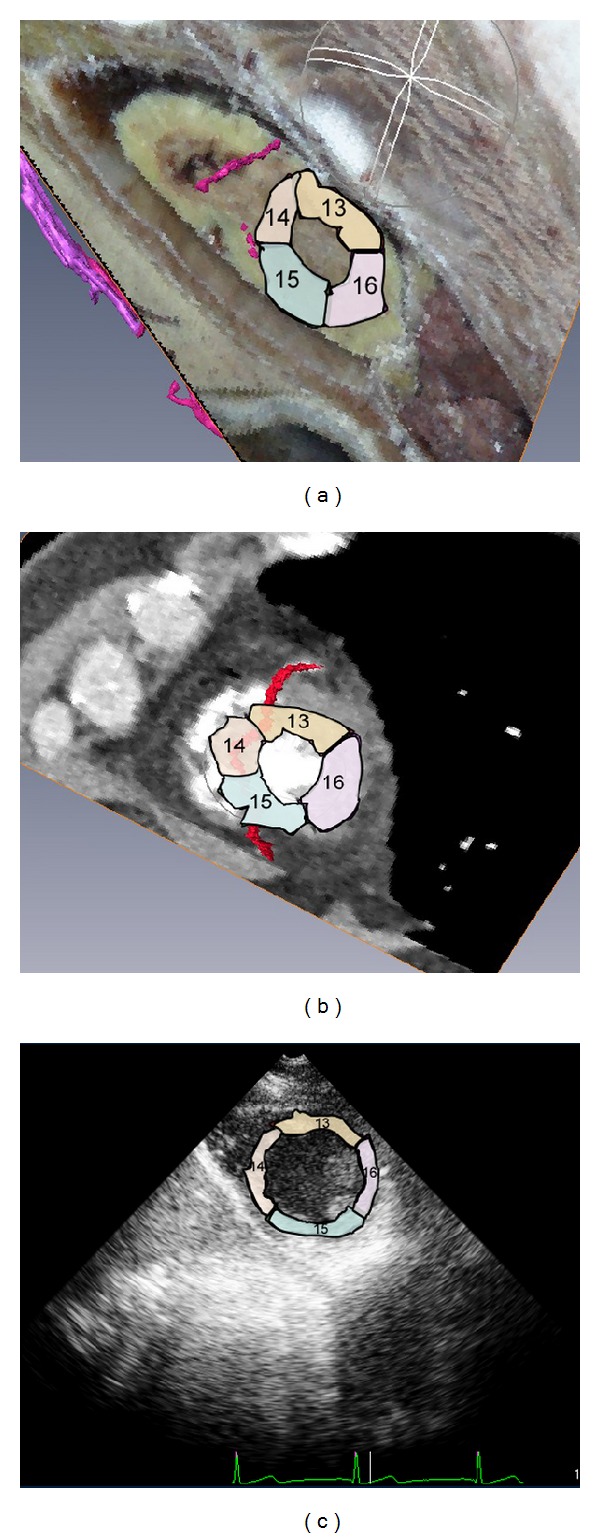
Short-axis sections of the left ventricular apex. (a) Fusion display of the CVH dataset and the coronary artery model. (b) Fusion display of the cardiac CT dataset and the coronary artery model. (c) Short-axis section of the left ventricular apex revealed by echocardiography.
